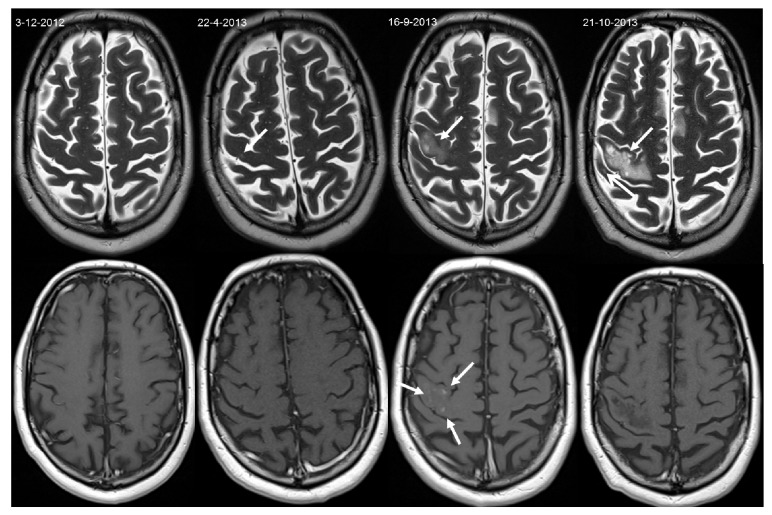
Axial T2-weighted (top row) and contrast-enhanced T1-weighted images (bottom row) obtained during Natalizumab treatment (3 December 2012), at the time of Natalizumab discontinuation (22 April 2013), at the time of PML-IRIS occurrence (16 September 2013), and after PML-IRIS treatment (21 October 2013). A small lesion suggestive of PML was already visible at the time of Natalizumab discontinuation (arrow). During Fingolimod treatment, this lesion progressed and changed to a PML-IRIS manifestation with slight mass effect and contrast enhancement (arrows) leading to posttreatment sequelae (arrow).
